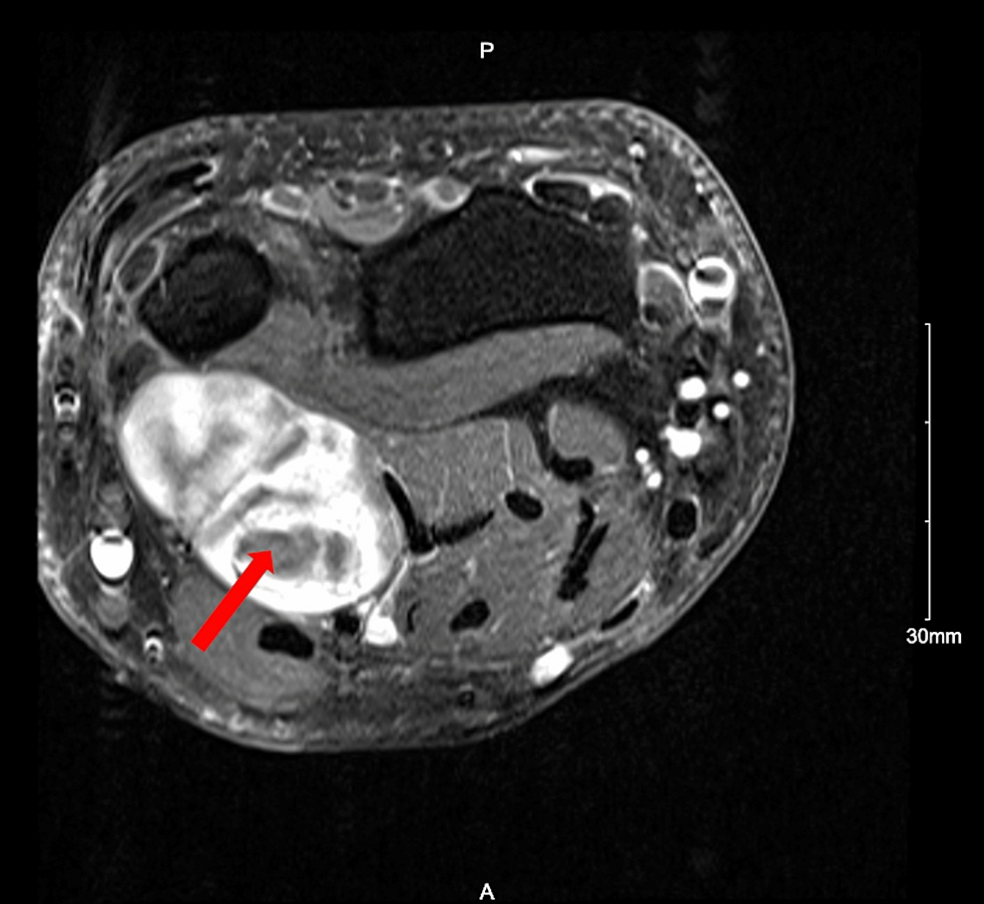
Axial T2 MRI of the left forearm showing the bilobed neural sheath tumor of the ulnar nerve in the distal 1/3 of the forearm. The mass is positioned between the flexor carpi ulnaris muscle and the flexor digitorum profundus and to a lesser extent flexor digitorum superficialis muscles. The mass terminates at the distal ulna with no extension into the region of Guyon's canal. Contrast administration showed heterogeneous consistency with areas of probable cystic change or internal necrosis. MRI, magnetic resonance imaging
